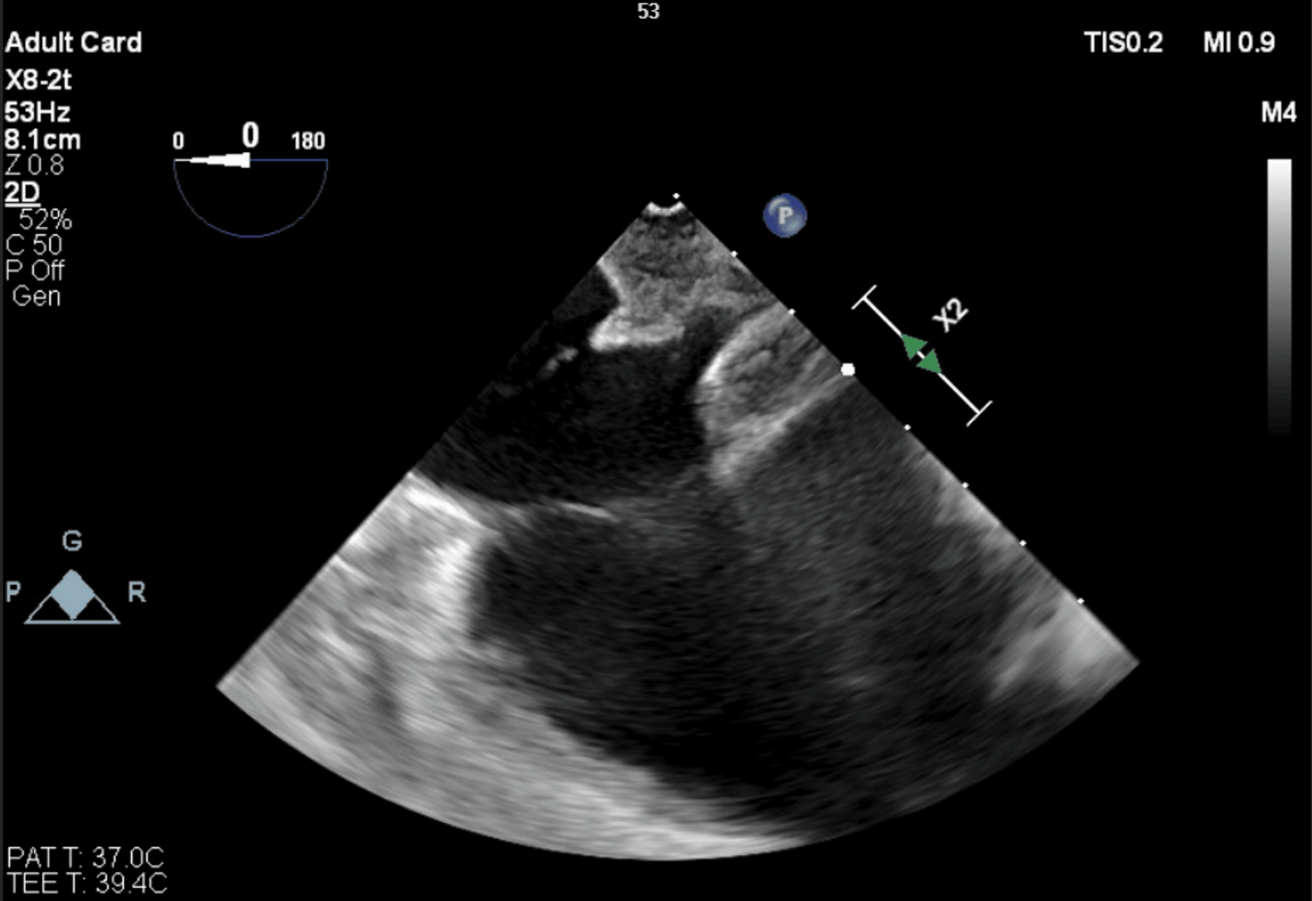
Repeat transesophageal echocardiogram (TEE) performed after the patient was found with a new pulmonary embolism, which no longer demonstrated previously visualized tricuspid valve vegetation.
